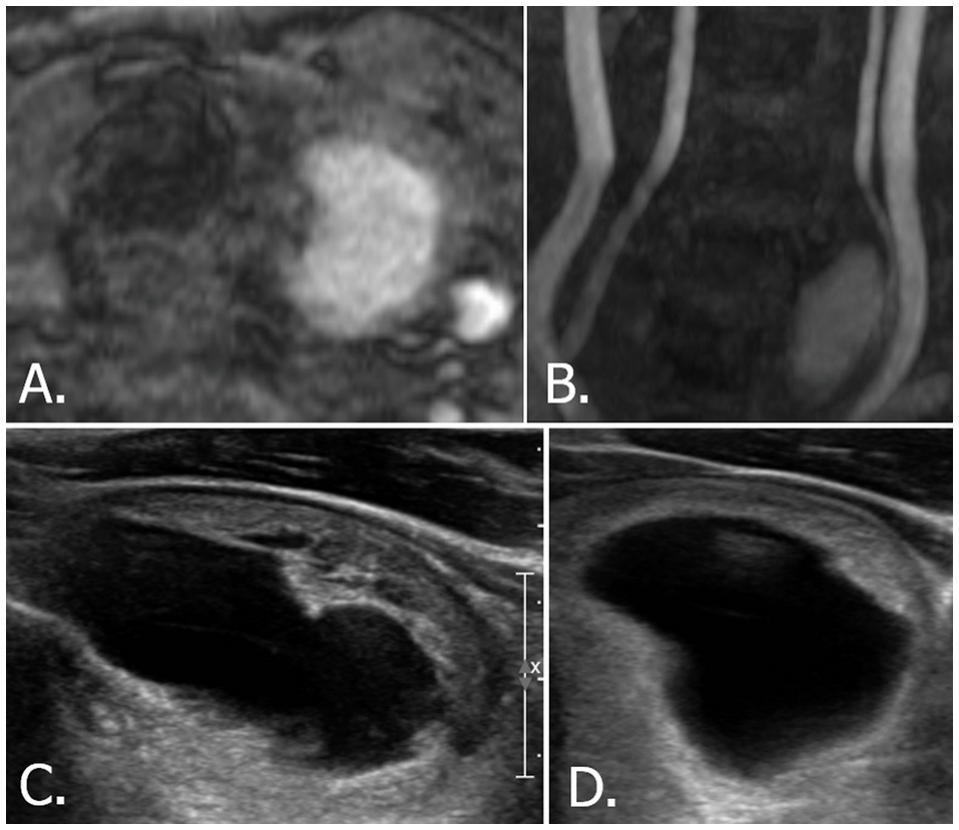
58-year-old male with left thyroid nodule. Source (A) and MIP (B) images of TOF-MR angiography show a totally hyperintense nodule in the left thyroid gland. Longitudinal (C) and transverse (D) US images of left thyroid reveals a smooth oval, predominantly cystic nodule with eccentric solid portion, which is classified as K-TIRADS category 3, ACR-TIRADS category 2, and EU-TIRADS category 3. MIP, maximum intensity projection; TOF-MR, time-of-flight magnetic resonance; US, ultrasound; K-TIRADS, Korean Thyroid Imaging Reporting and Data System; ACR-TIRADS, ACR Thyroid Imaging Reporting and Data System; EU-TIRADS, European Thyroid Imaging Reporting and Data System.
